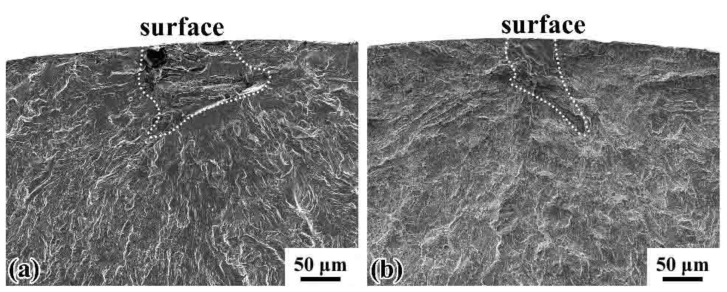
Typical fracture morphologies of surface crack initiation by ultrasonic fatigue test: (a) *R* = −1, *σ*_a_ = 263 MPa, *N*_f_ = 1.55 × 10^6^ cycles; (b) *R* = 0.3, *σ*_a_ = 227 MPa, *N*_f_ = 1.83 × 10^6^ cycles.
